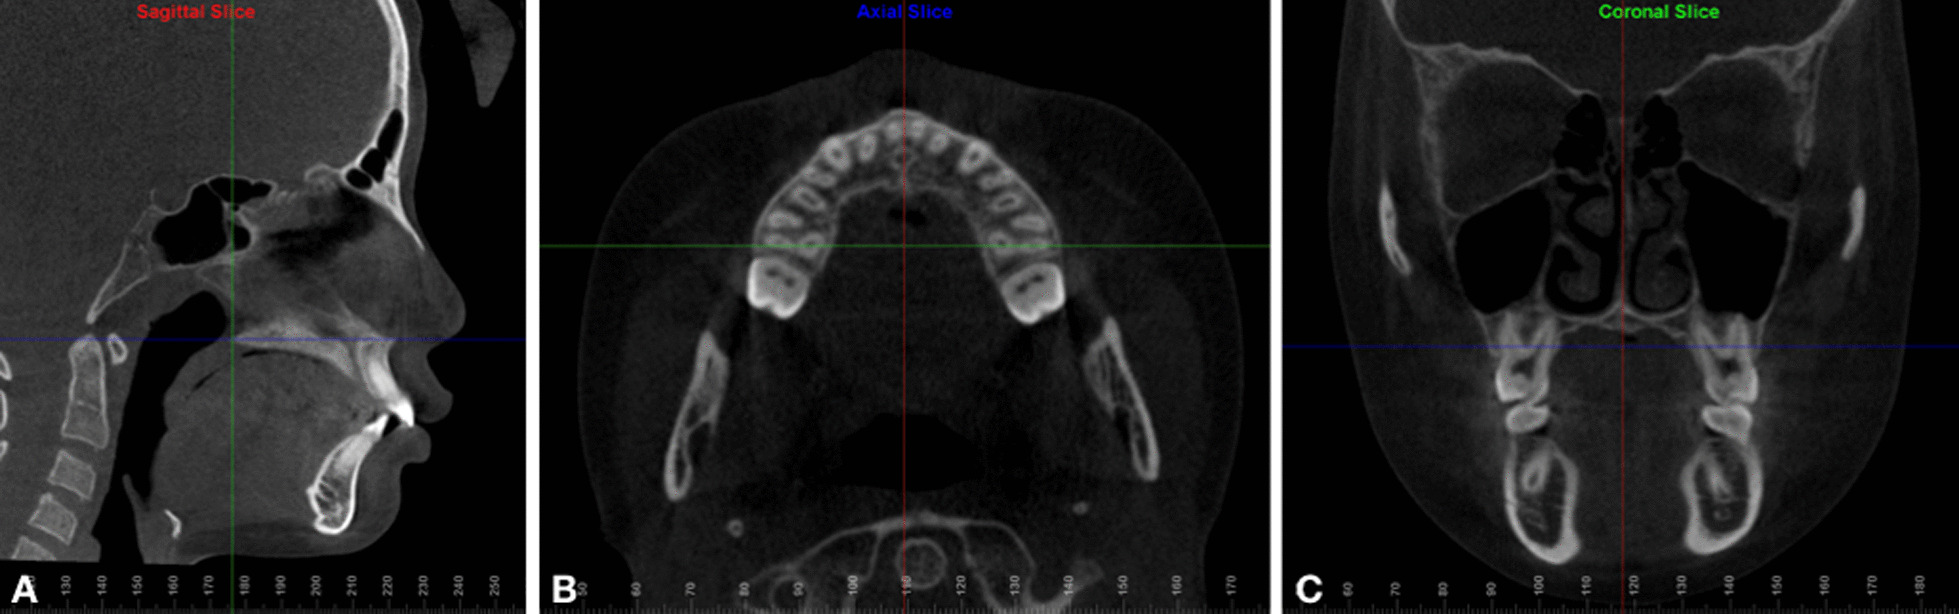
Re-orientation of CBCT images. Reoriented as parallel to the palatal plane (a sagittal section), passing through the root apices of both maxillary first molars (b axial section), and parallel to the hard palate (c coronal section)
